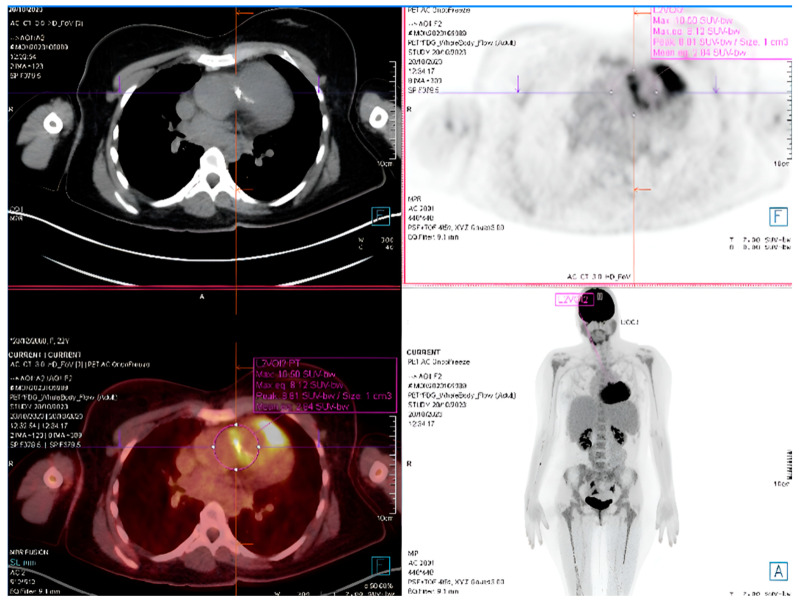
PET/CT of a patient with tetralogy of Fallot and infective endocarditis of the pulmonary conduit.
